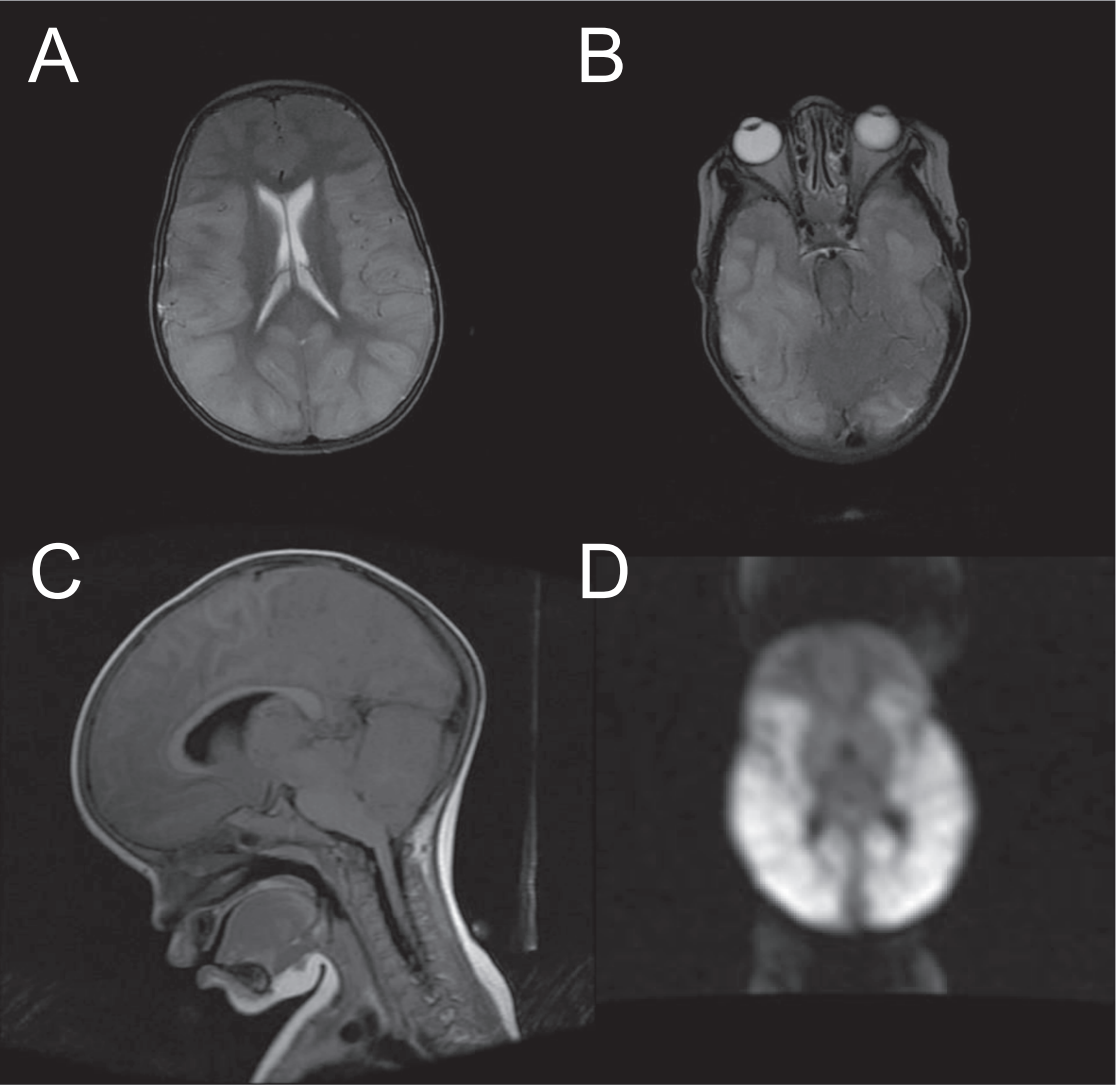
MRI of paediatric patient with fatal CM from Malaŵi presenting severe brain swelling. Admission axial T2 scan (TR 3140, TE 123) of a 4 years old male child with fatal retinopathy-positive CM performed at Queen Elizabeth Central Hospital in Blantyre, Malaŵi (A). Severe brain swelling is observed, with complete loss of sulcal markings. (B) shows a lower slice depicting severe brainstem compression resulting from the swelling. A mid-sagittal T1 Flair image (TR 2311, TE 26, ET 2) of the same patient reveals the complete effacement of all basilar cisterns and the 4^th^ ventricle, as well as severe brainstem compression (C). Finally, (D) shows potential diffuse restricted diffusion in the cerebral cortex and white matter. The child succumbed from respiratory failure 3 hours after these images were obtained.
